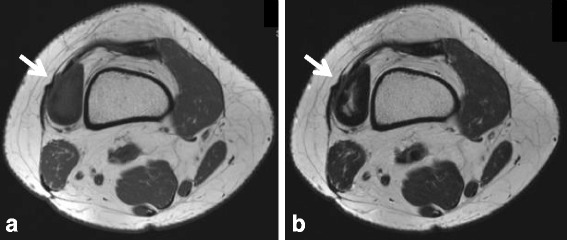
T1- and T2-weighted MR images of Case 2. a On T1-weighted images, the mass (arrow) is isointense compared to muscle. b On T2-weighted images, the mass (arrow) has high signal intensity in the center of the mass, and the surrounding area has low signal intensity
